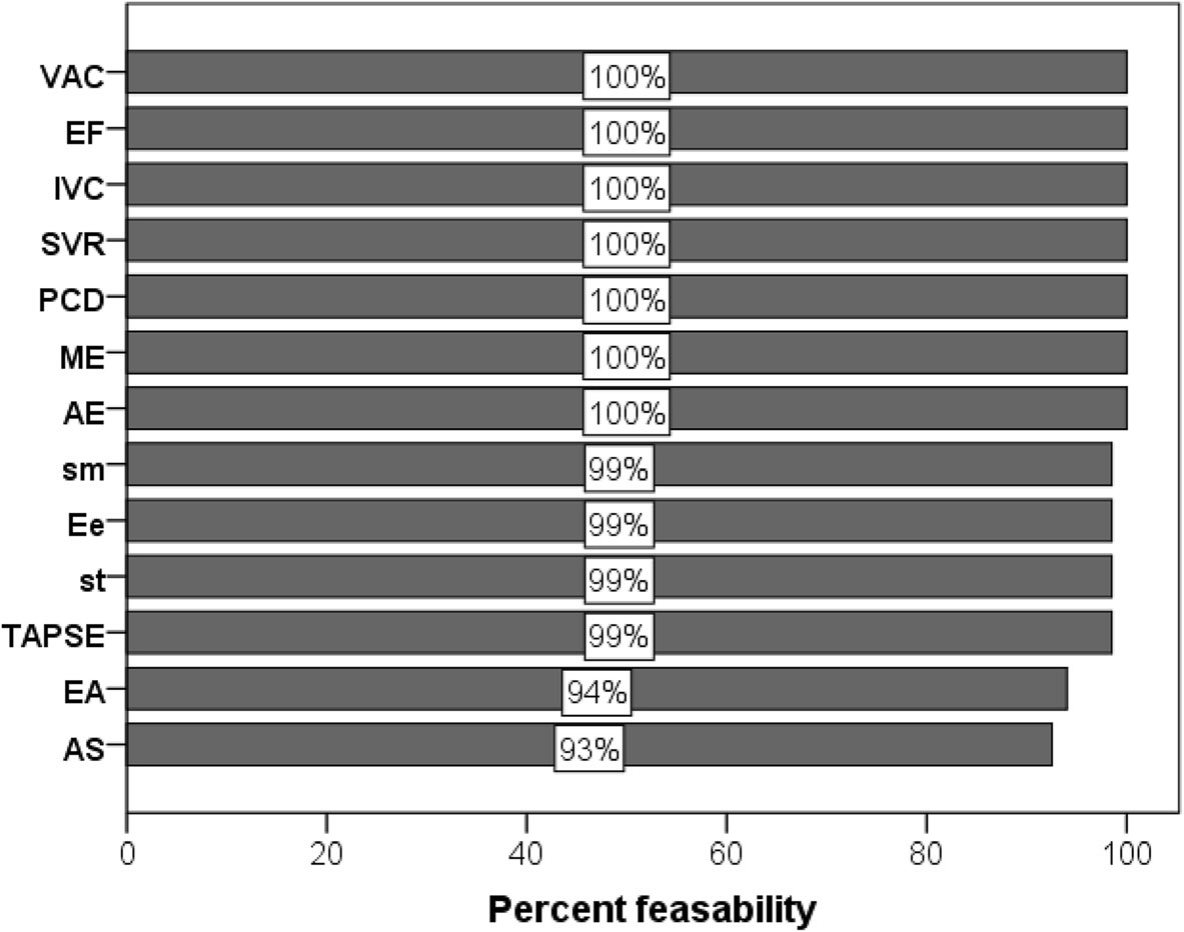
Feasibility of measuring echocardiographic parameters in critically ill patients with coronavirus-19 sepsis. VAC, ventricular-arterial coupling; EF, LV ejection fraction in %; IVC, maximal diameter of inferior vena cava in mm; SVR, systemic vascular resistance in mmHg L^−1^ min; ME, LV end-systolic maximal elastance in mmHg mL^−1^; AE, end-systolic arterial elastance in mmHg mL^−1^; sm, tissue Doppler peak systolic wave at lateral mitral annulus in cm s^−1^; Ee, ratio of early pulsed-wave Doppler to early tissue Doppler diastolic wave velocity at the lateral mitral valve annulus; st, tissue Doppler peak systolic wave at tricuspid lateral annulus in cm s^−1^; TAPSE, tricuspid annulus plane systolic excursion in mm; EA, ratio of early to late diastolic wave velocity at the mitral valve; AS, absolute values of global LV longitudinal peak systolic strain in %; PCD, pulmonary circulatory dysfunction
